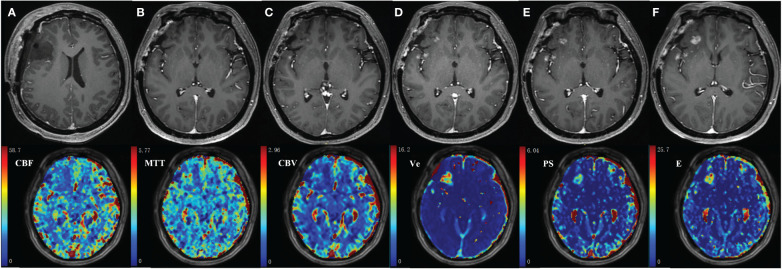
35-year-old female with WHO grade 4 glioma of the right frontal lobe. Postoperative pathology confirmed the enhanced lesion as radiation necrosis (RN). Row 1: follow-up MR examinations after surgery showed the presence and evolution of enhancing lesion. (A) MR image after the first surgery; (B) 30 months after surgery; (C)31 months after surgery; (D)33 months after surgery; (E)35 months after surgery; (F)37 months after surgery. Row 2: parametric maps of blood flow CBF, mean transit time MTT, fractional volume of intravascular space CBV, fractional volume of interstitial space V_e_, vessel permeability PS, and extraction ratio E as derived using DP model.
